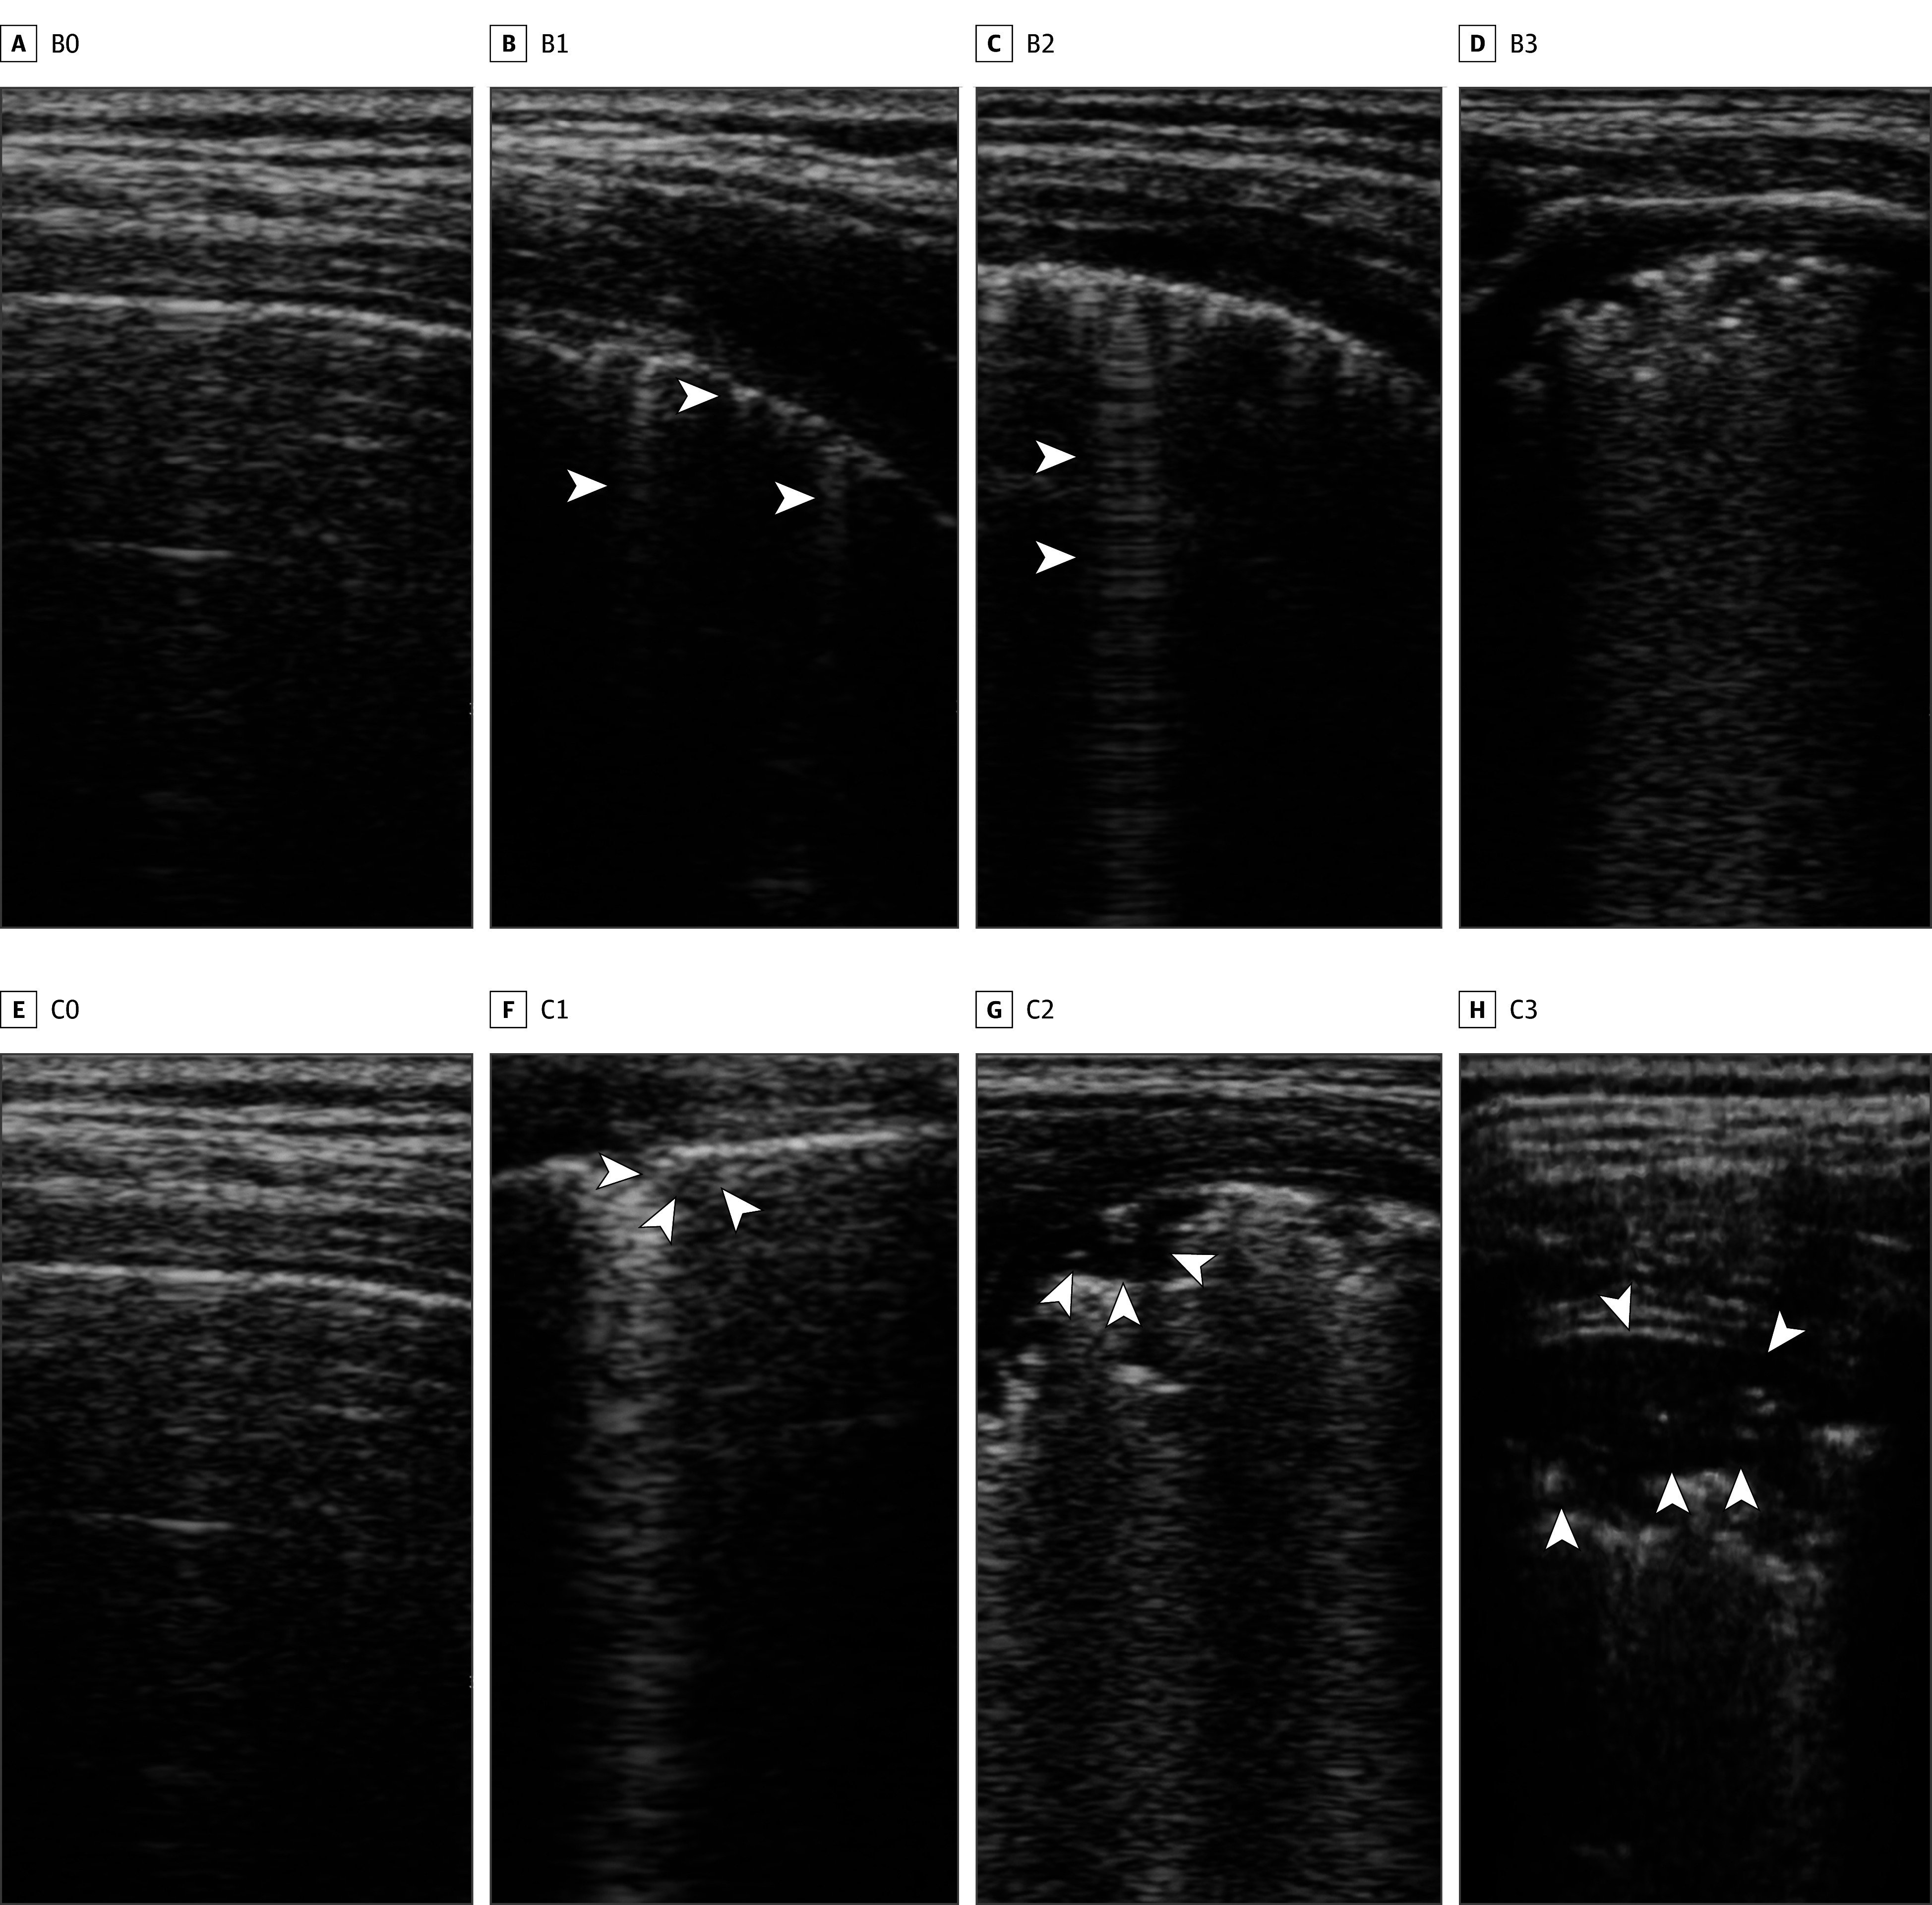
Lung Ultrasound Findings of Consolidations and B-Lines The degree of B-lines was divided into 4 grades as follows: B0, fewer than 3 isolated B-lines; B1, multiple well-defined B-lines; B2, multiple coalescent B-lines; and B3, white lung. The degree of consolidation was divided into 4 grades as follows: C0, no consolidation; C1, minimal juxtapleural consolidations; C2, small-sized consolidations; and C3, large-sized consolidations. Arrowheads indicate pathologic findings.
